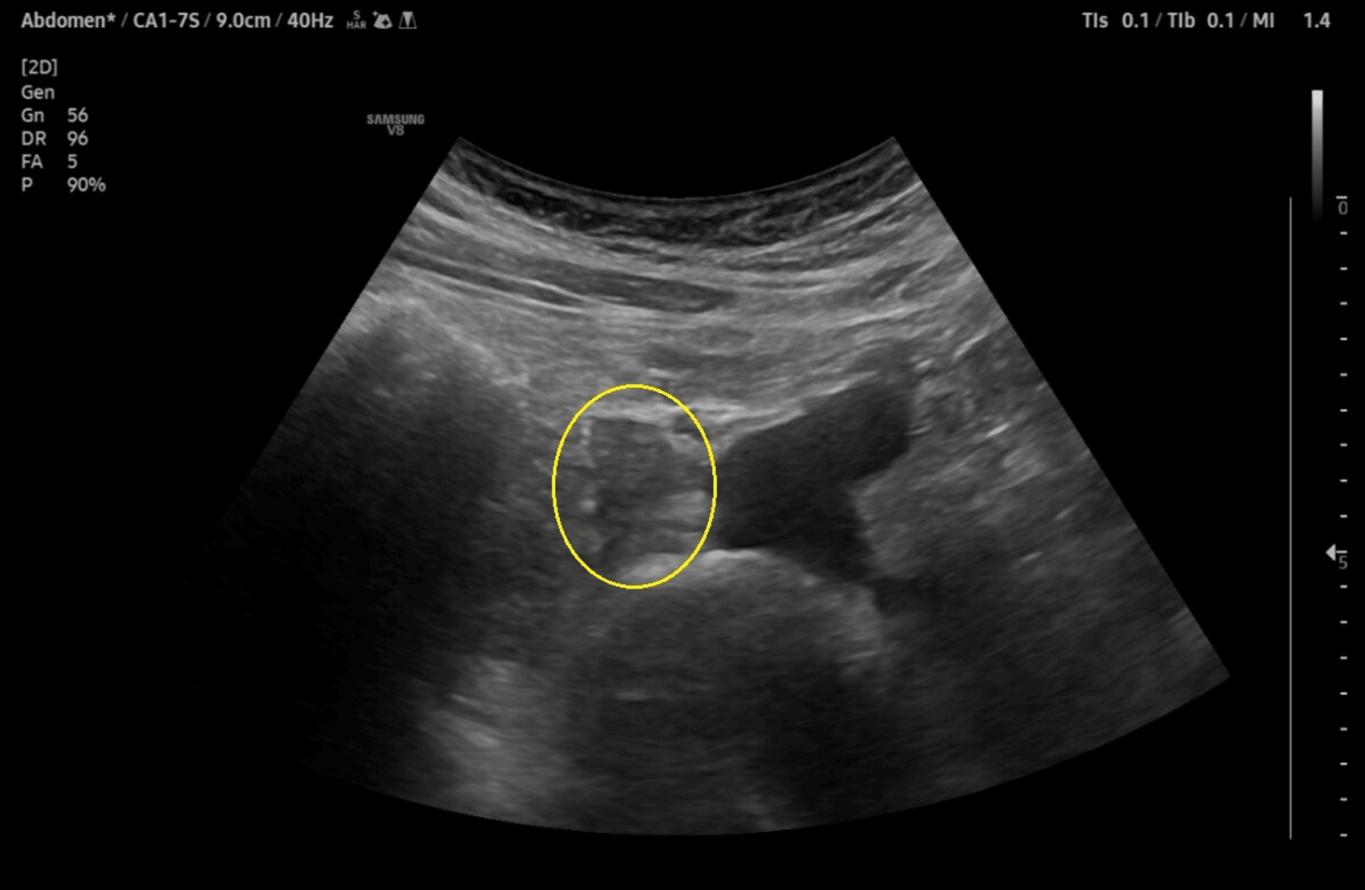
Abdominal ultrasound scan image, showing an irregular shaped soft tissue density lesion (eclipse) in the right lower quadrant, measuring about 16 x 20 mm. Differential diagnosis includes inflammatory changes (panniculitis).
